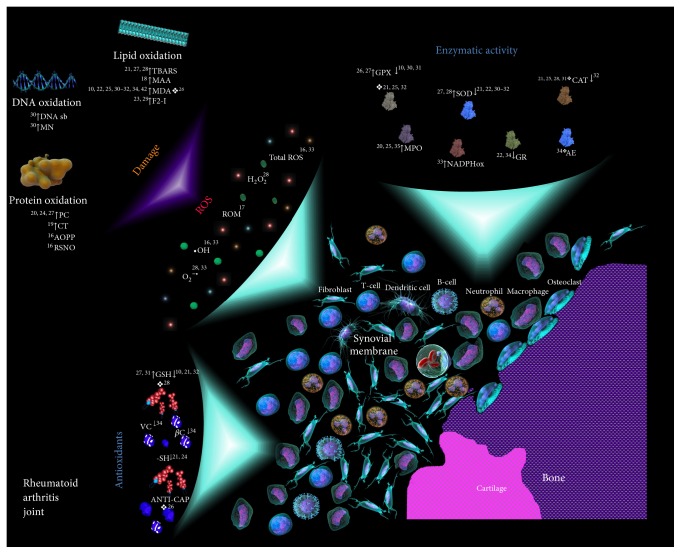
Oxidants/antioxidants biomarkers and oxidative damage found in joints and blood of RA patients. The literature references pertaining to the indicated phenomena are provided in the scheme. Under oxidative stress conditions, the joints and blood of patients with RA show high concentrations of free radicals, mainly ROS (gray), which induce DNA, proteins, and lipids damage through different mechanisms. The nonenzymatic antioxidant response (blue square), in general, is diminished. The enzymatic activity (including enzymatic antioxidant response) shows variability. AE: arylesterase, ANTI-CAP: total antioxidant capacity, AOPP: advanced oxidation protein products, CAT: catalase, CT: 3-chlorotyrosine, DNA sb: DNA strand breaks, F2-I: F2-isoprostane, GPx: glutathione peroxidase, GR: glutathione reductase, GSH: reduced glutathione, H_2_O_2_: hydrogen peroxide, MAA: malondialdehyde-acetaldehyde, MDA: malondialdehyde, MN: micronucleus, MPO: myeloperoxidase, NADPH ox: reduced nicotinamide adenine dinucleotide phosphate oxidase, O_2_
